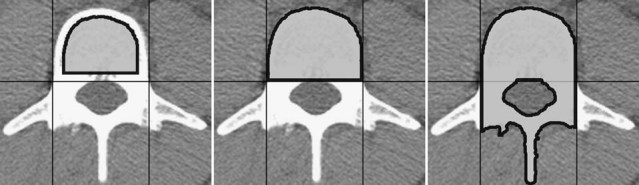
Regions of interest for the CT images. Left to right: cancellous bone region; isolated vertebral body; vertebral body including the posterior elements.
